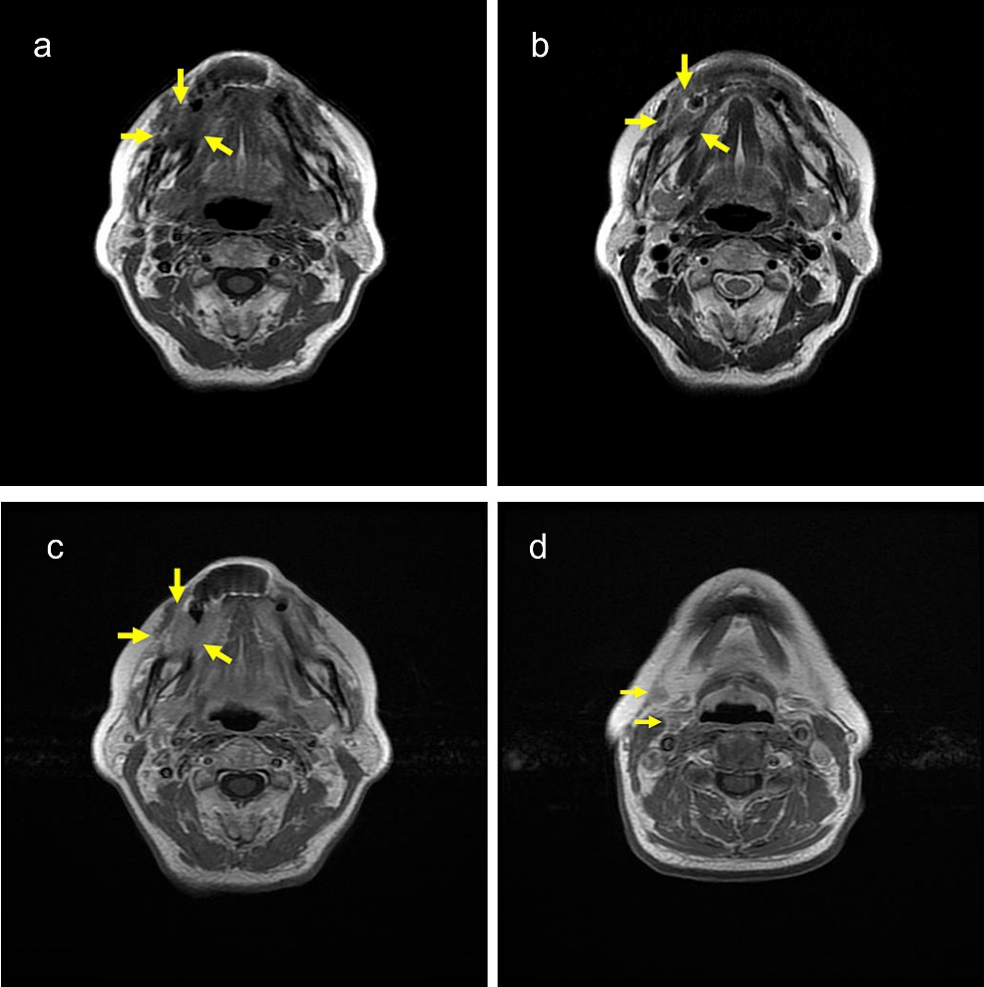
Axial MRI of the head and neck MRI showed an irregular tumor in the lower right gingiva. The tumor showed lower intensity on a T1WI (yellow arrow) (a) and intermediate intensity on a T2WI (yellow arrow) (b). A gadolinium-enhanced T1WI, a uniform enhancing tumor extended to the mandible bone (yellow arrow)(c). A gadolinium-enhanced T1WI shows two small lymph nodes of the right neck (yellow arrow) (d).
